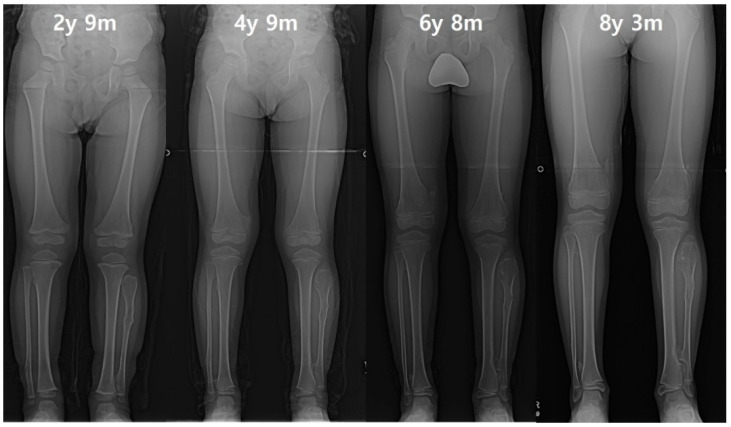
The serial radiographs of a male patient demonstrated progression of valgus deformity on the left ankle. Despite exostoses in bilateral legs, valgus angulation of the left ankle accompanied by shortening of the fibula was observed, while the neutral alignment was maintained on the right ankle. In the last measurement, the left tibial length was also about 9 mm shorter than the right tibia.
